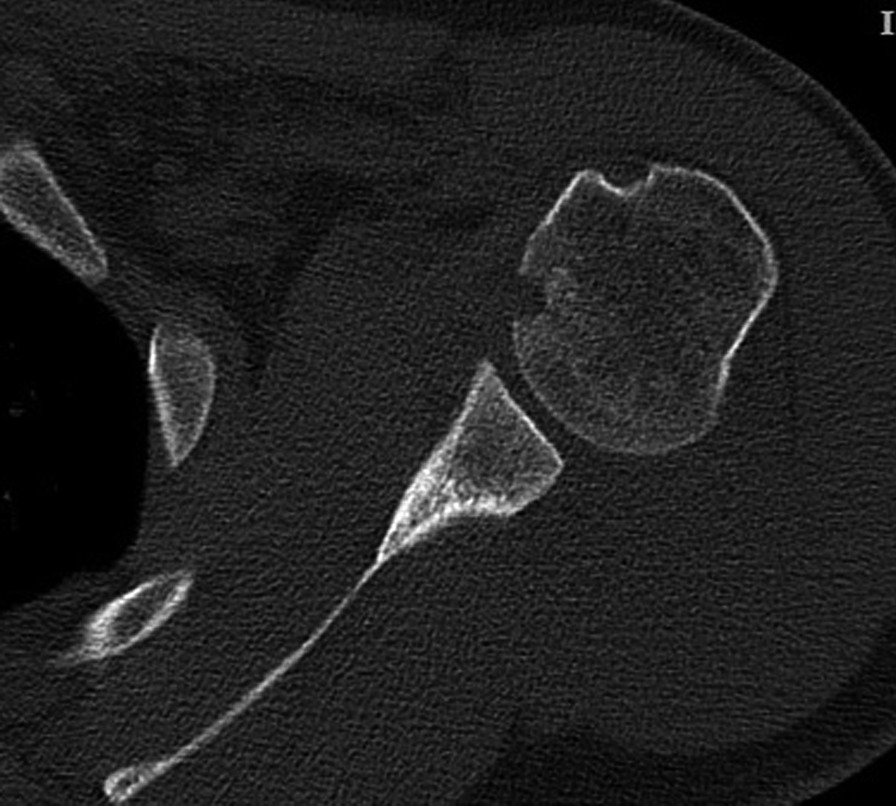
Small impacted fracture in the anteromedial of the humeral head indicative of the reverse Hill–Sachs lesion in three-dimensional computed tomography (3D CT) scan
